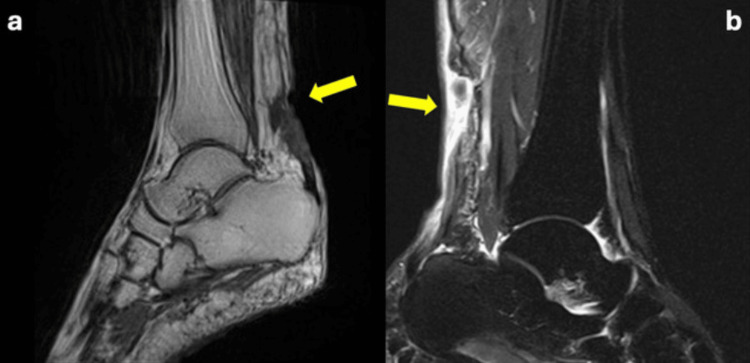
T1-weighted image of the right ankle (a) and T2-weighted image of the left ankle (b) revealing a bilateral Achilles tendon rupture (yellow arrows)
